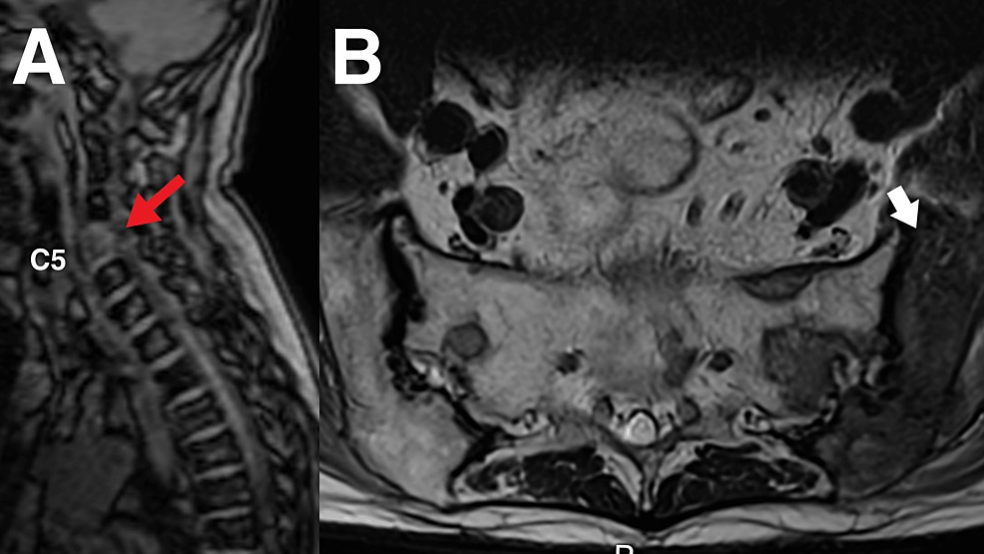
Magnetic resonance imaging (MRI) of the lumbar spine A) An equivocal lesion is seen at C5 vertebral body (red arrow) on initial localizer radiographs. B) several ill-defined bone lesions are seen on the left of the ilium (white arrow) in this MRI that indicate a diagnosis of strongly suspected bone metastases.
